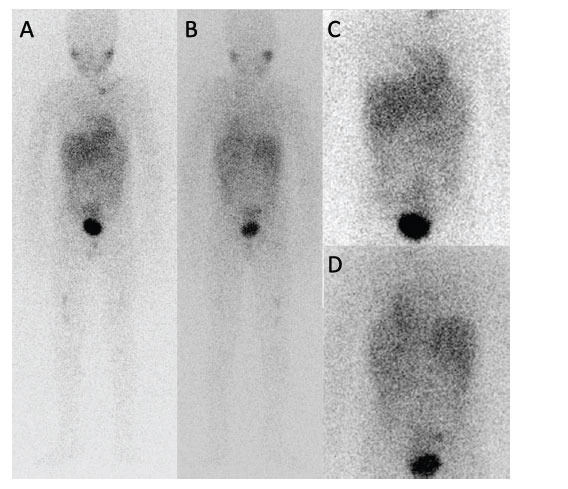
The I123-MIBG scan of Case 1 reveals metastatic bone uptake in the left and right distal femur, the right proximal tibia, and left clavicle. Conversely, there was no abnormal uptake in the abdomen, which does not align with the findings of the Ga68-DOTA peptide PET/CT scan. (A). Anterior whole-body scan (B). Posterior whole-body scan (C and D). Anterior and posterior thoracoabdominal static images.
